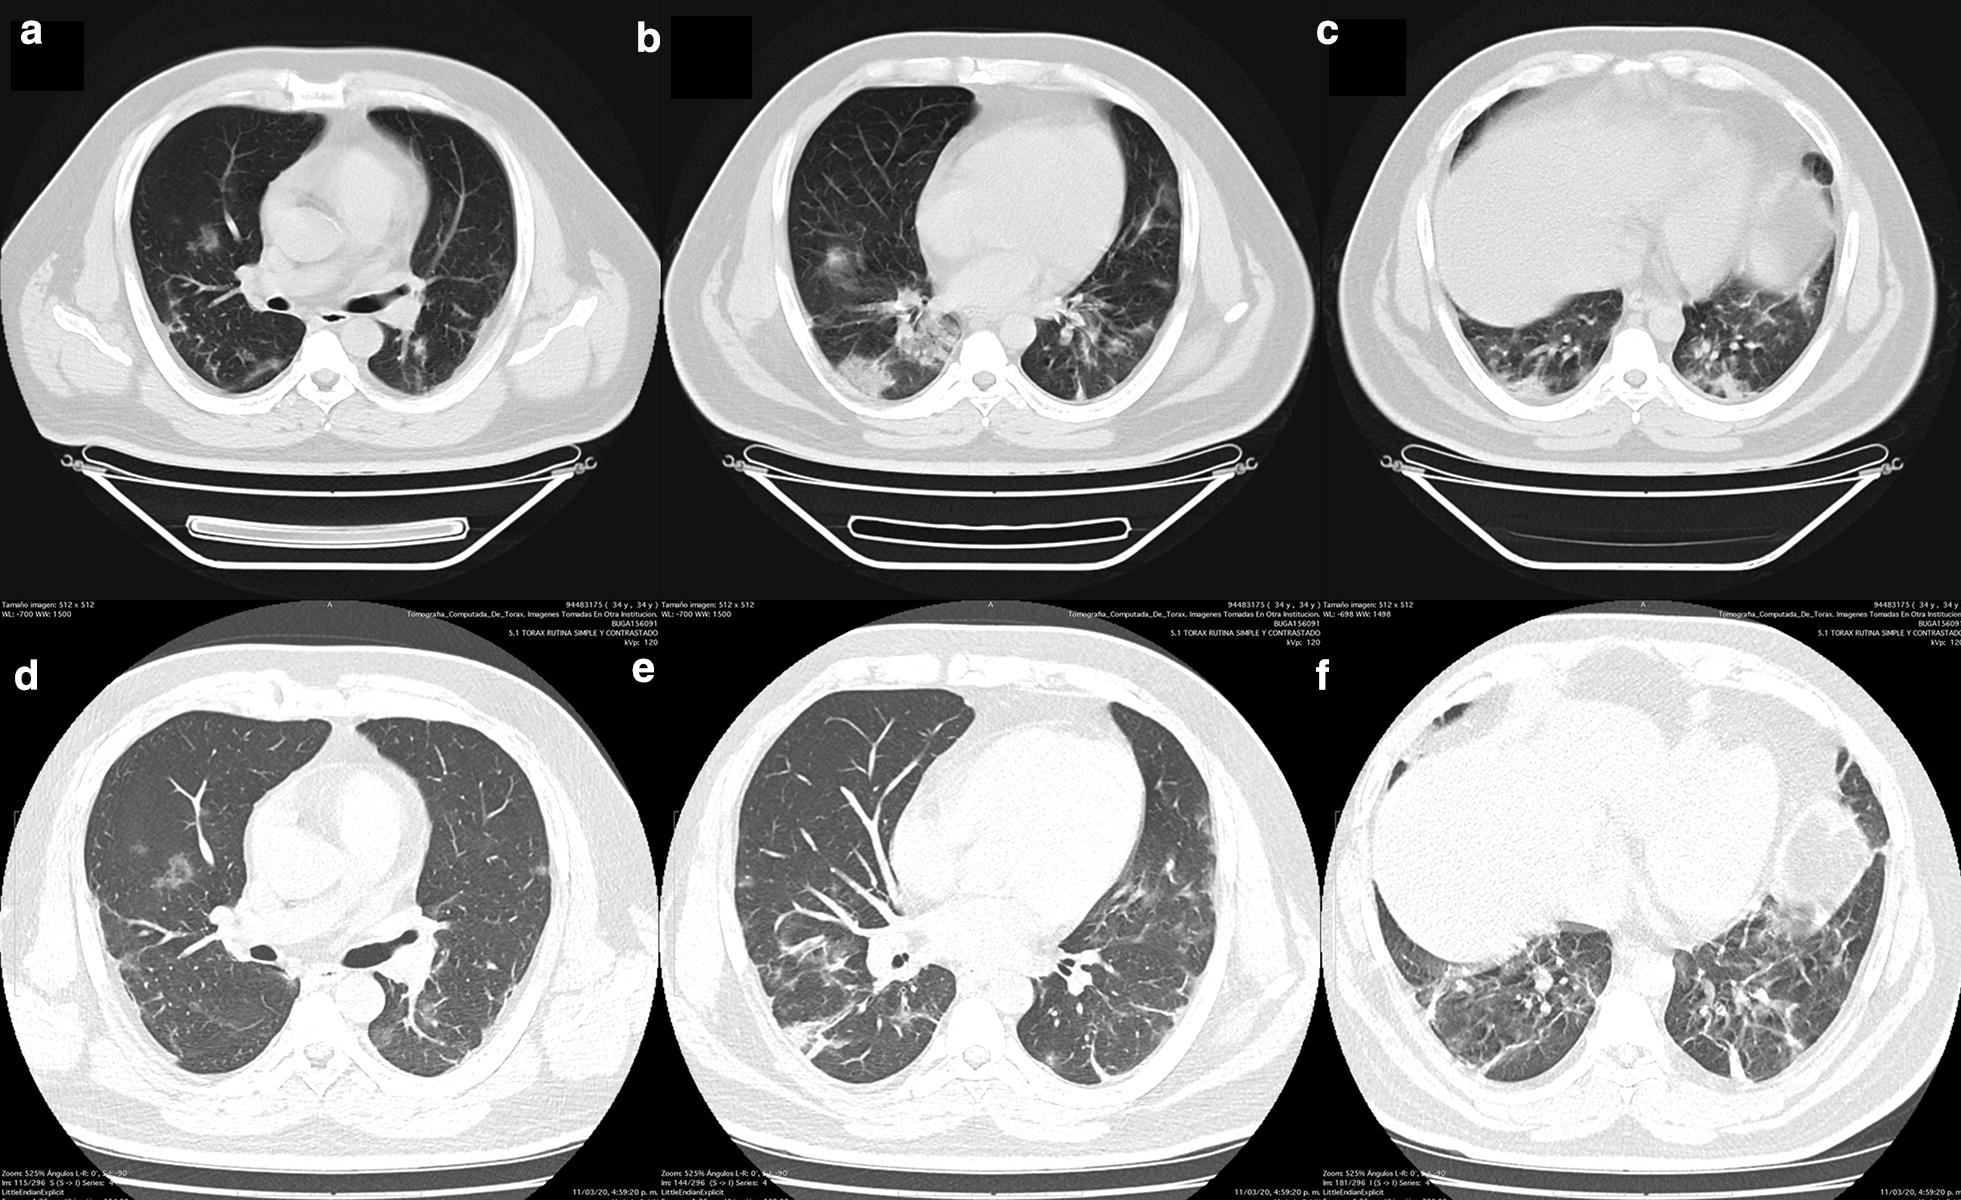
a–c Chest computed tomography on admission showed peripheral ground-glass opacities with a partial occupation, and also complete consolidation in mid- and lower-third of the thorax. d–f Chest computed tomography, 4 days later, showed peripheral ground-glass opacities with partial occupation improving, the reversed halo sign is observed, with decreasing in the size of the consolidations
